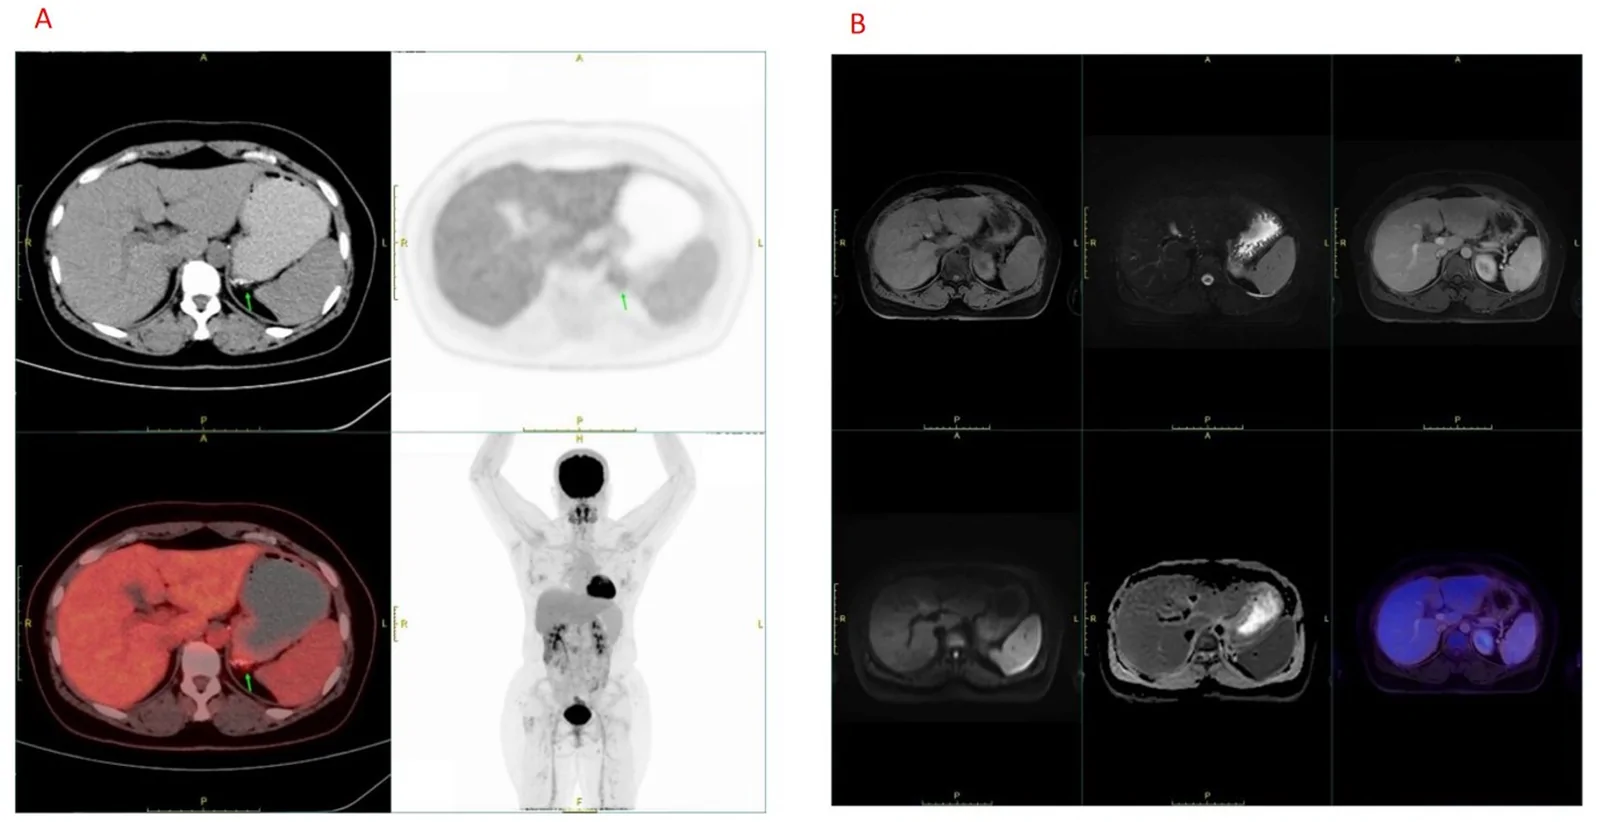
Postoperative follow-up functional imaging examinations at the early postoperative stage. (A) Follow-up PET/CT: Only postoperative benign changes were observed in the left adrenal region, without detectable abnormally increased radiotracer uptake suggestive of residual or recurrent lesions. (B) Follow-up PET/MRI: The left adrenal gland was surgically resected. No abnormal imaging signals or pathological radiotracer accumulation were identified within the surgical bed.
